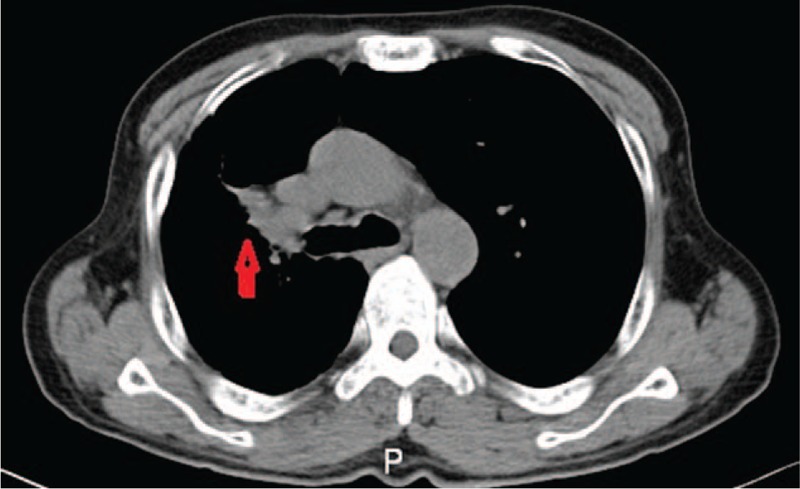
Chest computed tomography (CT) after the fourth cycle of chemotherapy indicates the lesion in the right lung.
